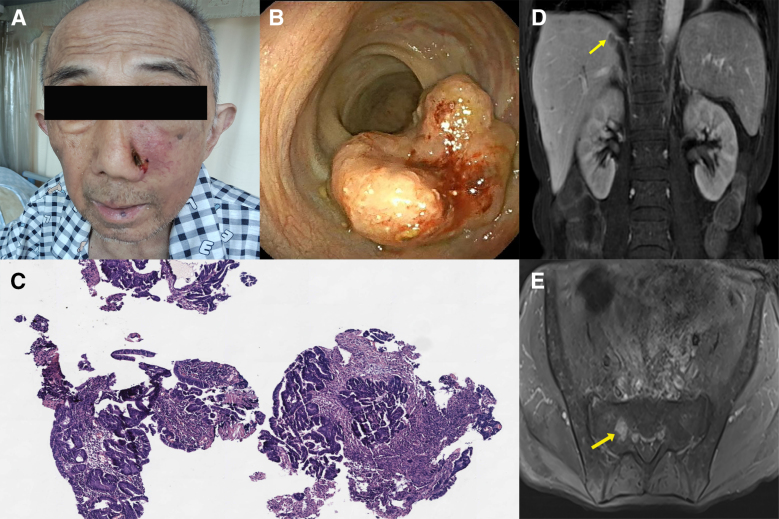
Physical examination findings and diagnostic evaluation of rectal adenocarcinoma. (A) A 0.5-cm sinus tract with purulent discharge on the left nasal ala, (B) colonoscopy revealed a cauliflower-shaped mass in the mid-rectum, (C) rectal biopsy showed moderately differentiated adenocarcinoma with atypical glandular formation (20×), (D) enhanced MRI revealed a metastatic lesion in the right sacrum, (E) enhanced MRI revealed a metastatic lesion in the right sacrum. MRI = magnetic resonance imaging.
